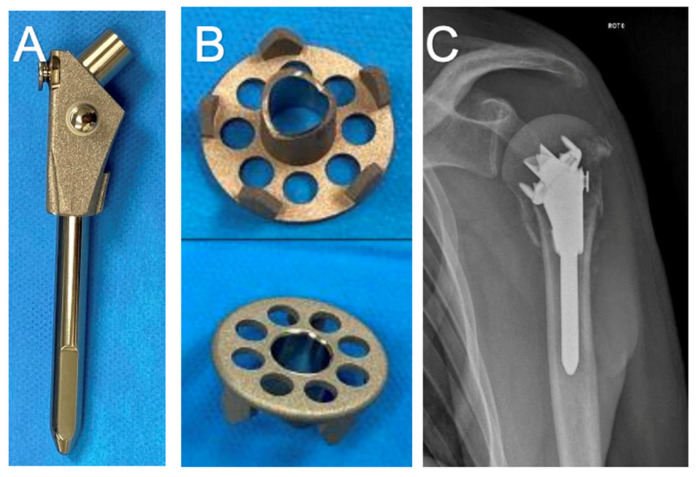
The Bilboquet implant: (A) JUST cementless stem (Evolutis, Briennon, France), (B) head staple component, (C) X-ray demonstrating Bilboquet implant.
